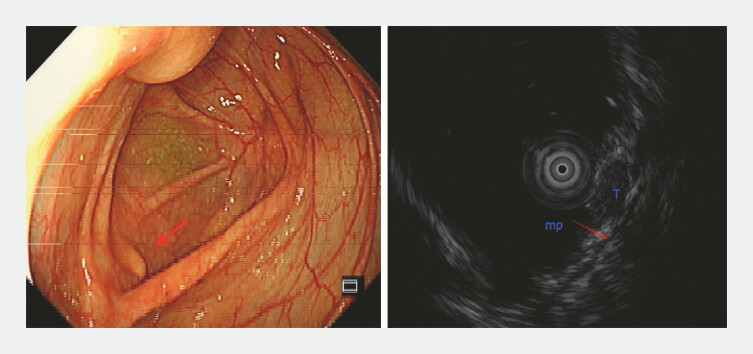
Yellow submucosal lesion (red arrow) confirmed by ultrasound to be located in the submucosal layer.
